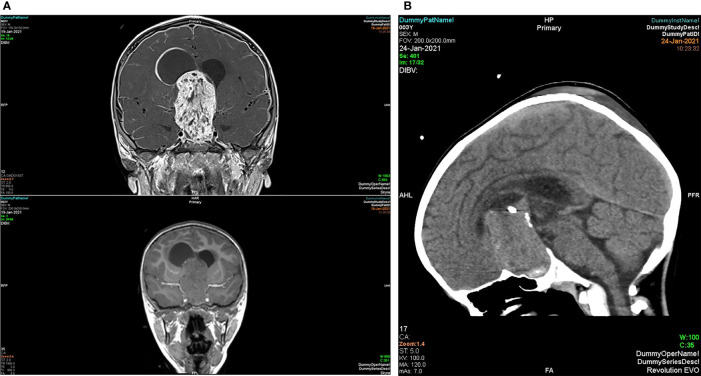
(A) MRI T1 with (top) and without (bottom) contrast coronal view of a giant craniopharyngioma with sellar and suprasellar solid enhancing parts; A large cystic component extends into the frontal horn of the right lateral ventricle. (B) A mid sagittal plane CT head of the same patient with evidence of sellar widening and peripheral calcification.
